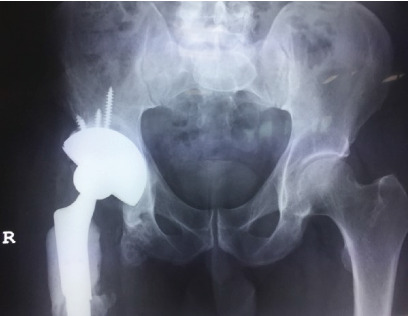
Pelvic X-ray post reimplantation procedure in 2017.
